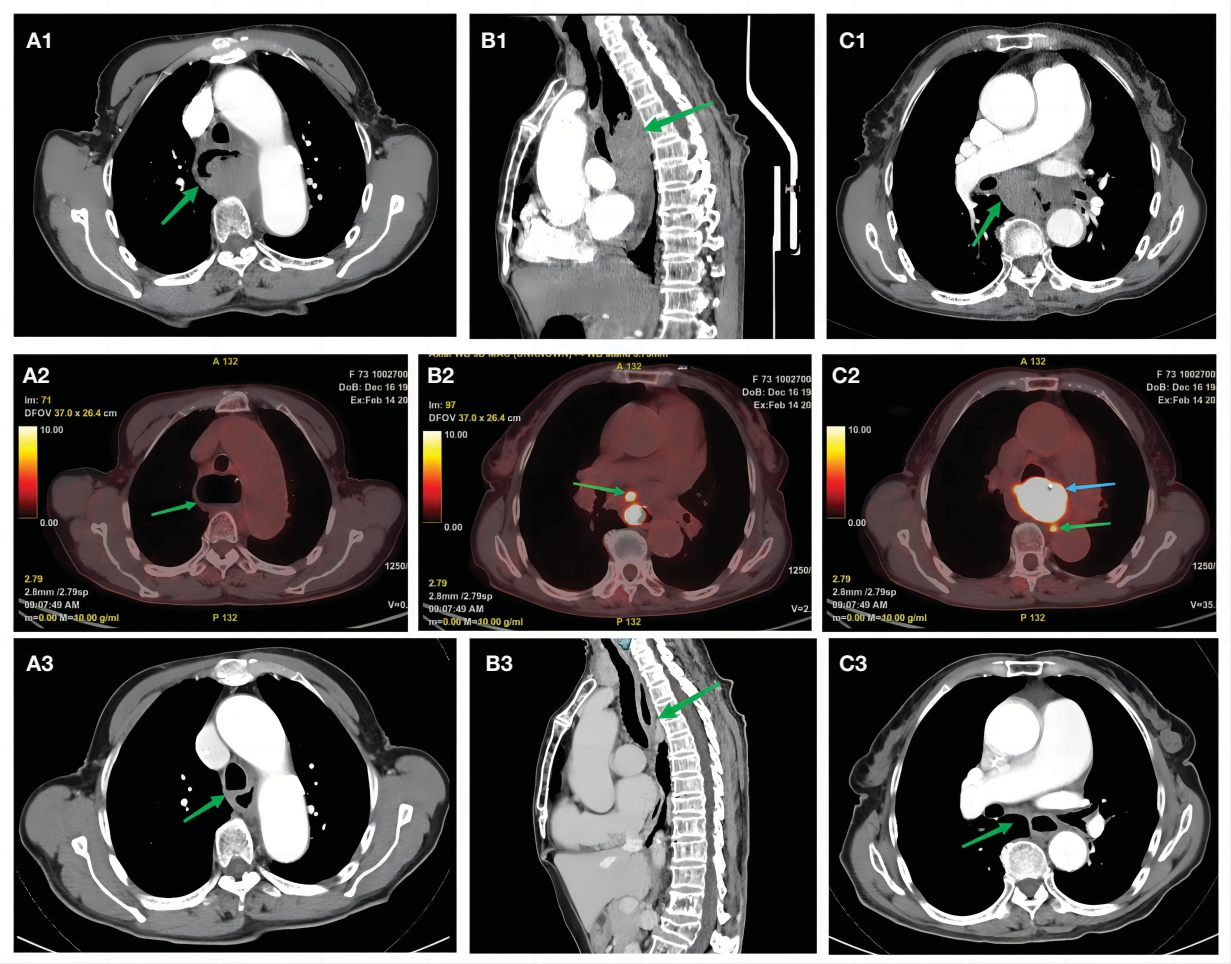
Pre-treatment examination: high-resolution computed tomography. (A1, B1) Green arrows indicate mid-esophageal occupations. (C1) Green arrow indicates an enlarged mediastinal lymph node (approximately 1.8 cm in diameter). Positron emission tomography/computed tomography examination. (A2) Green arrow shows dilated esophagus. (B2) Green arrow indicates mediastinal multiple lymph nodes with increased FDG metabolism. (C2) Green arrow indicates right paraesophageal hiatus lymph node with increased FDG metabolism, considered metastatic. (C2) Blue arrow indicates middle esophageal occupancy with increased FDG metabolism. Post-treatment examination: high-resolution computed tomography scan. (A3, B3) Green arrows indicate significantly shrunken esophageal cancer lesions compared with the previous scan (February 6, 2023). (C3) Green arrows indicate shrunken lymph nodes. FDG, fluorodeoxyglucose.
